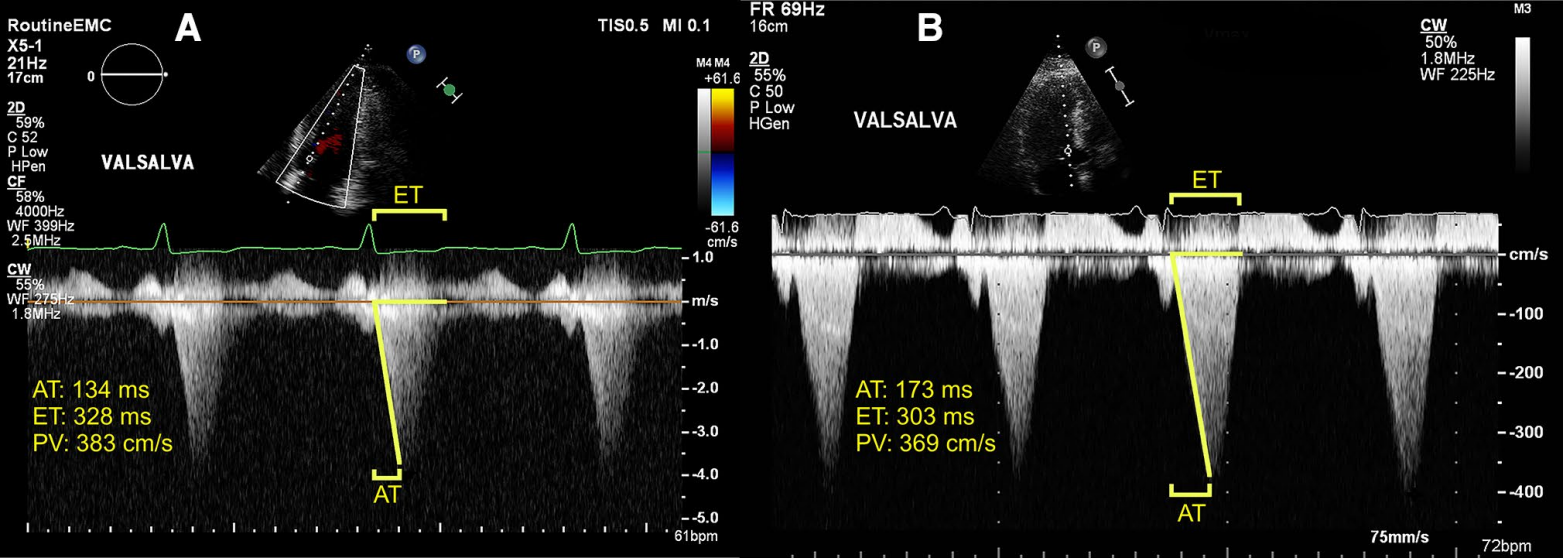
Examples of continuous-wave Doppler tracings used for acceleration time measurement. a apical five chamber (zoomed) recording of 54-year-old asymptomatic man. b apical three chamber recording of 59-year-old man with complaints of exertional dyspnea and (near-)syncope (New York Heart Association class III). Peak left-ventricular outflow tract gradients during provocation were relatively similar, but acceleration time was distinctly different between subjects. Intervals for acceleration time and left-ventricular ejection time are represented as yellow brackets
